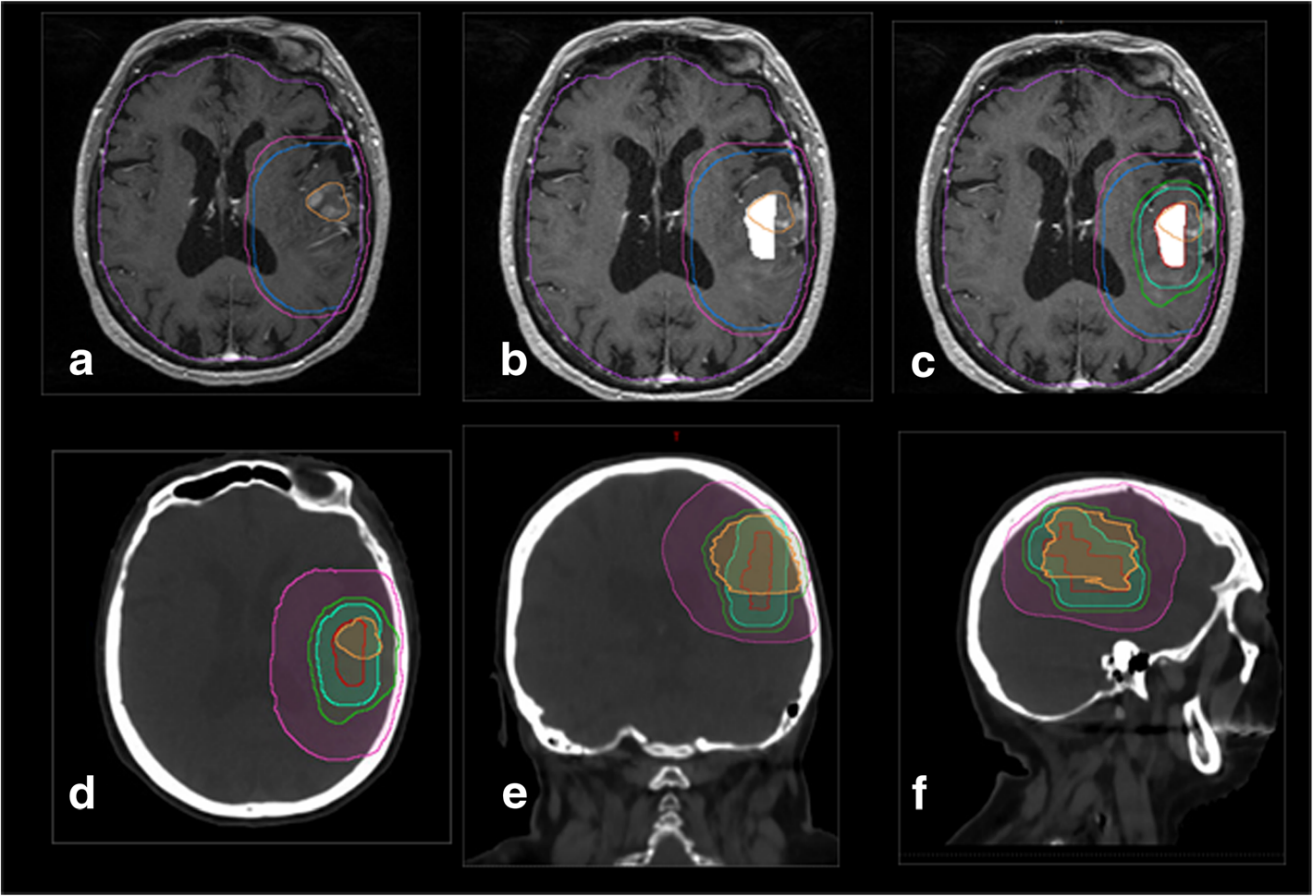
a-delineated GTV1(orange), CTV1 (blue), PTV1 (pink) on T1gado MRI, 4b MRSI map, 4Bc GTV1, CTV1, PTV1 GTV2(red), CTV2(cyan), PTV2 (green) 4d resulting volumes on axial view (d), frontal view (e) sagittal view (f)
